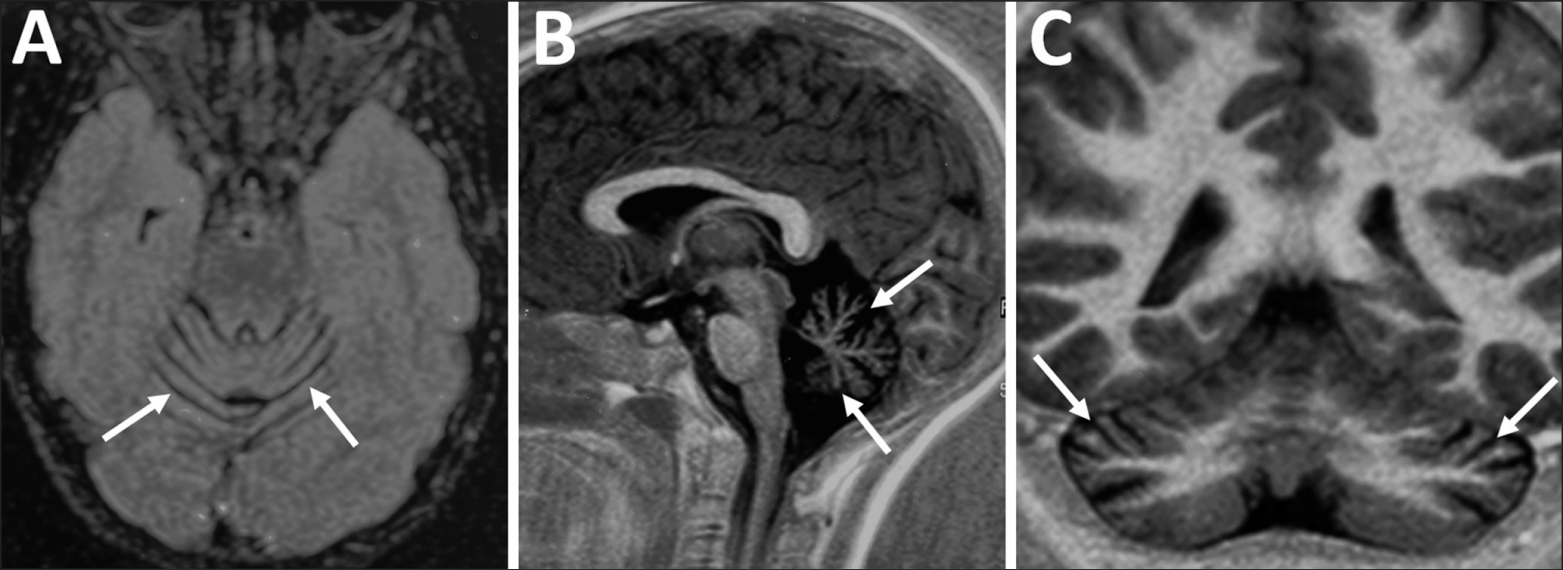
Axial Flair (panel A), sagittal T1-weighted (panel B) and coronal T1-weighted (panel C) brain magnetic resonance imaging showing cerebellar atrophy (white arrows).
